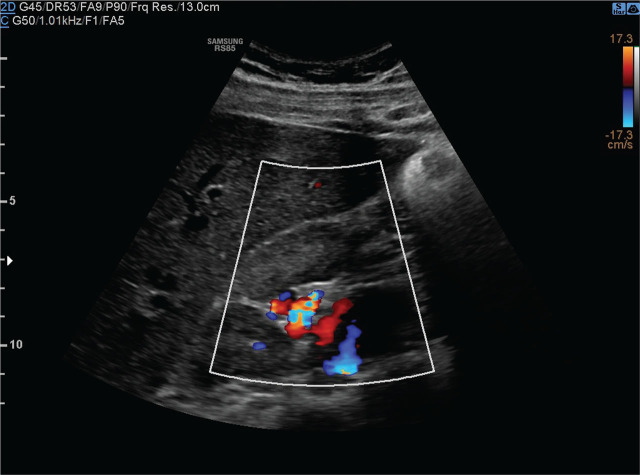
Abdominal ultrasound with power doppler shows blood flow within the right kidney cyst - peripheral circulation.
